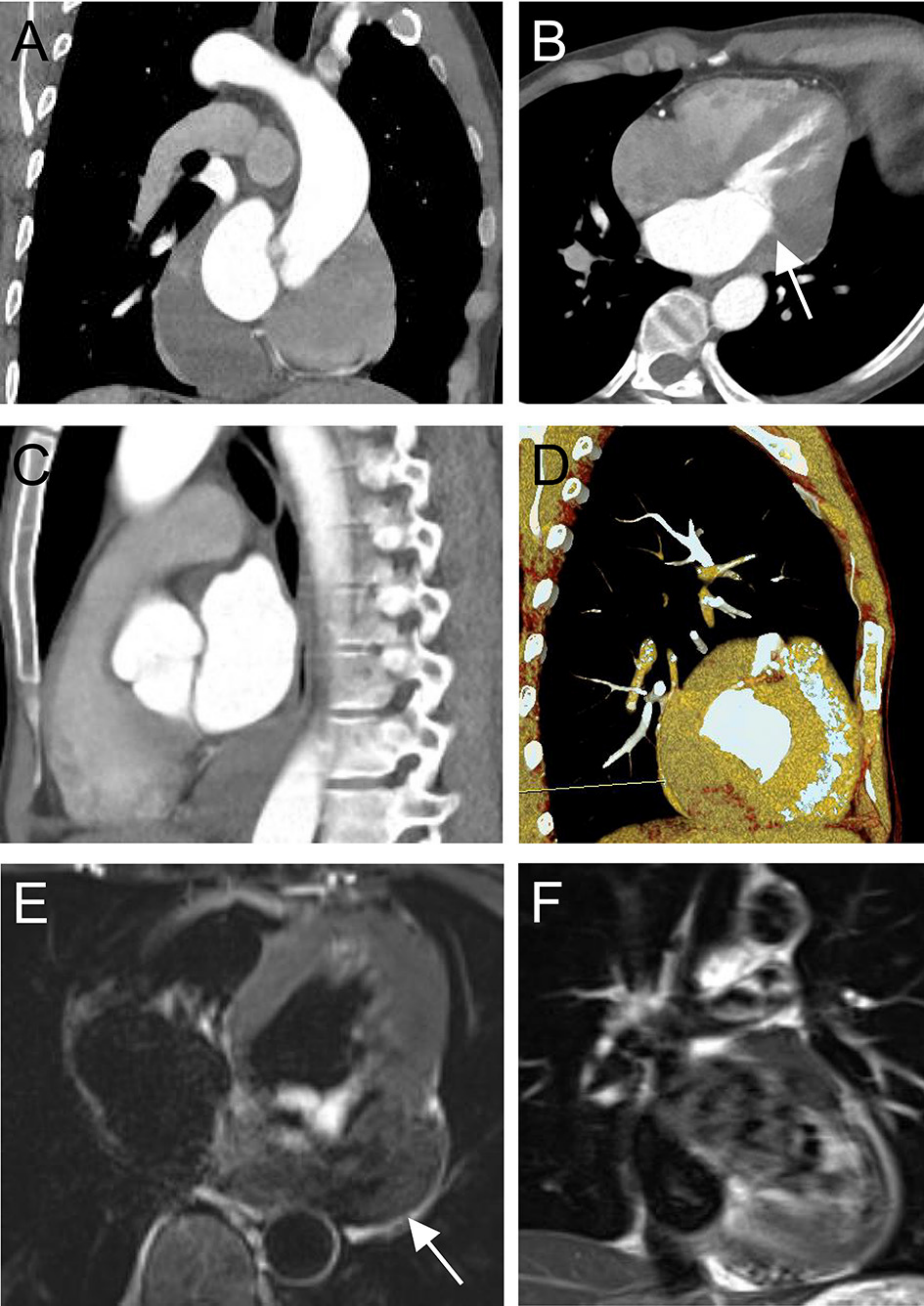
Computed tomography pre-operatively in coronal view (A), four-chamber view (B), sagittal view (C), three-dimensional volume-rendered image, and (D) shows a tumor-like structure located in the left atrioventricular groove (arrow) with a fountain sign from the posterior wall of the left atrium to the abnormal mass. Cardiac magnetic resonance imaging (E,F) pre-operatively showing a heterogeneous mass with the posterior wall of the left ventricle involved.
